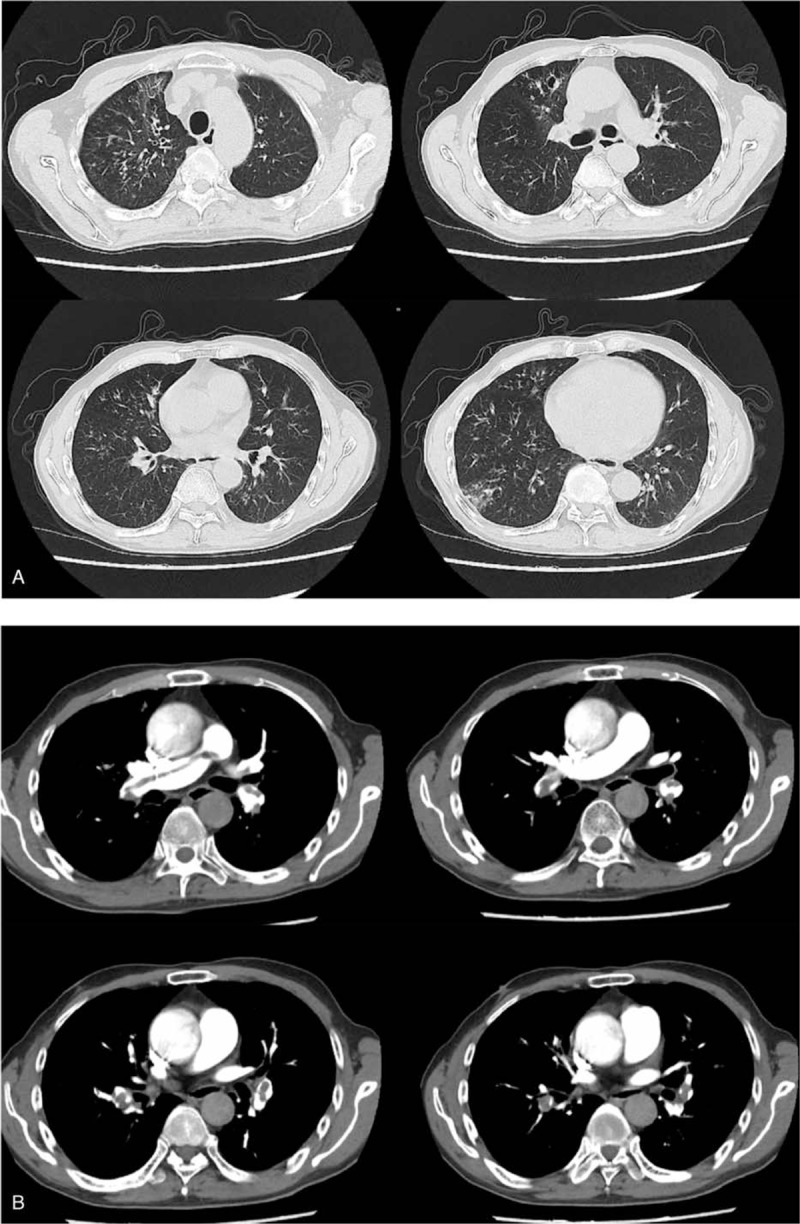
A. HRCT of the chest showing pulmonary infiltrates; B. Chest angiography showing filling defects in the main pulmonary artery and both branches of the pulmonary artery. HRCT = high-resolution computed tomography.
